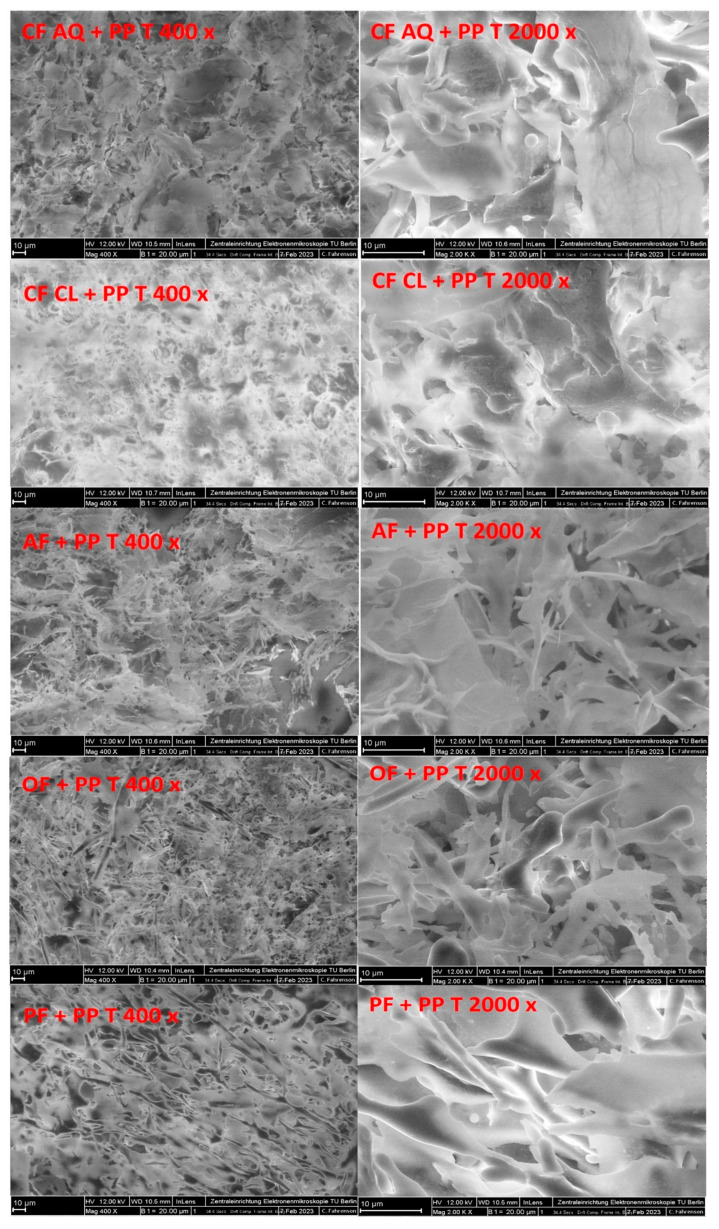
SEM images (400× and 2000× magnifications, scale bar 10 µm) of untreated (UT) and ultrasound-treated (T) pea protein and fiber samples. (PP—pea protein; CF AQ—citrus fiber AQ; CF Cl—citrus fiber Cl; AF—apple fiber; OF—oat fiber; PF—pea fiber).
